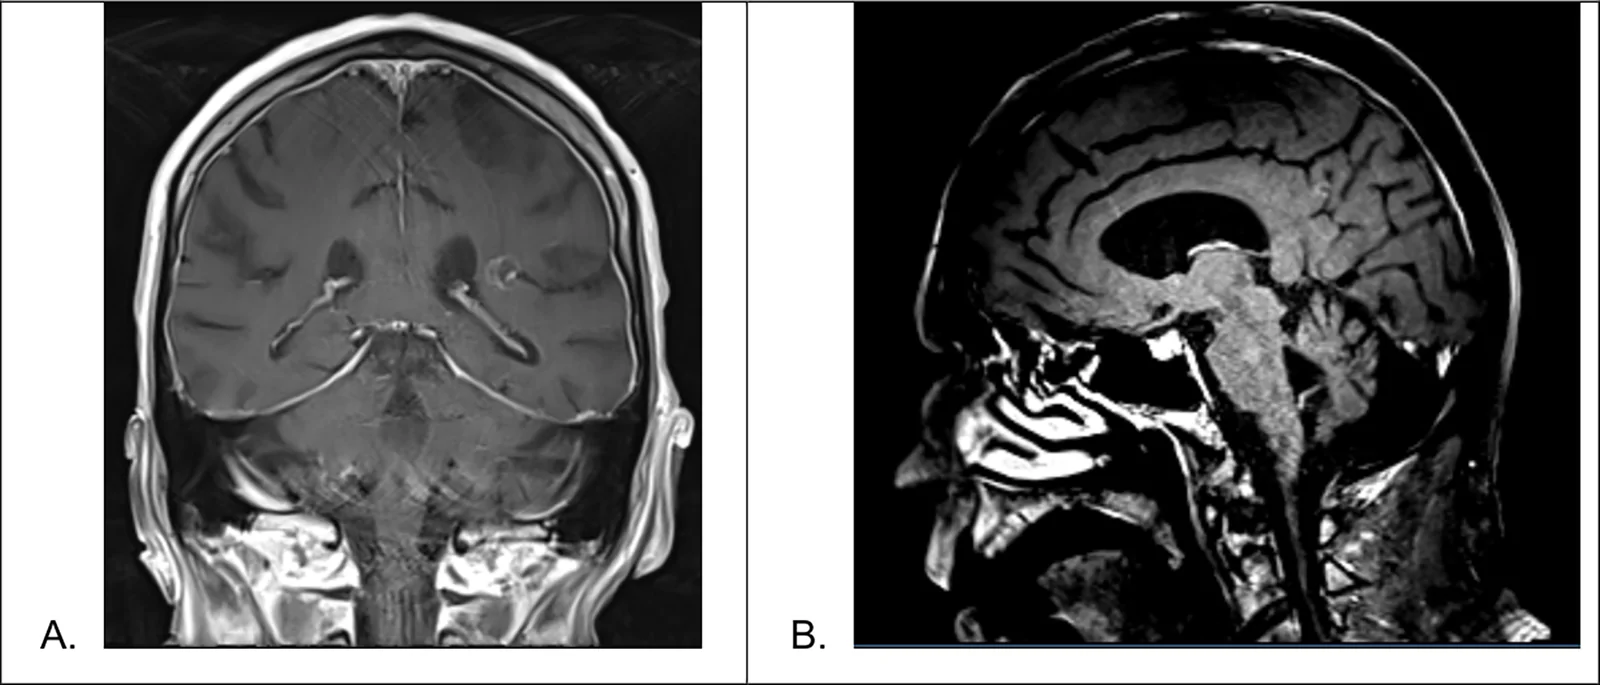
MRI of head. Two lesions were visible on the MRI. (A) Small rim-enhancing left posterior insular-perisylvian lesion with eccentric targetoid morphology. (B) Tiny rim-enhancing precuneus left parietal lesion.
